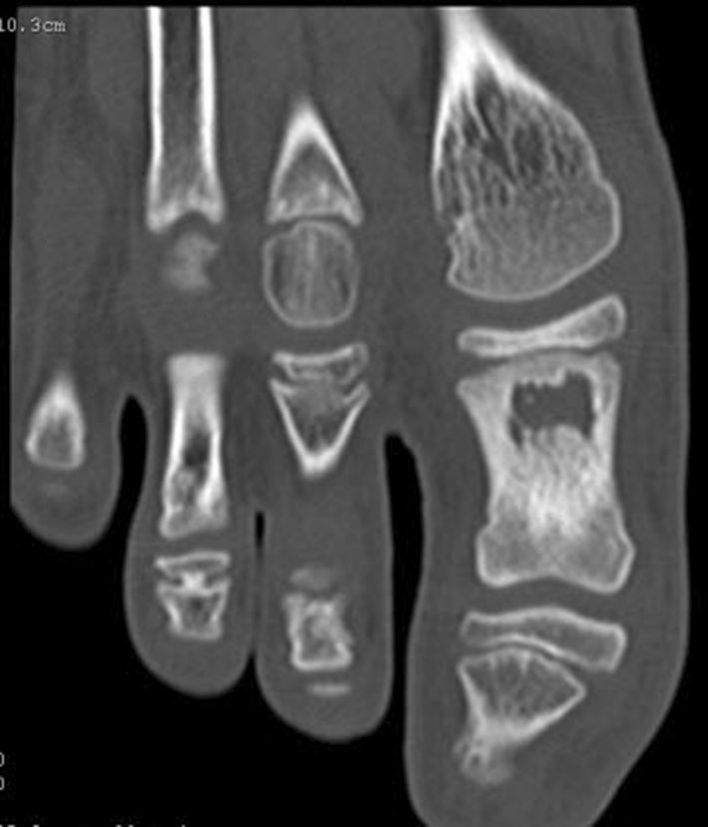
X-ray image showing lytic changes of osteomyelitis in the proximal phalanx of the big toe
